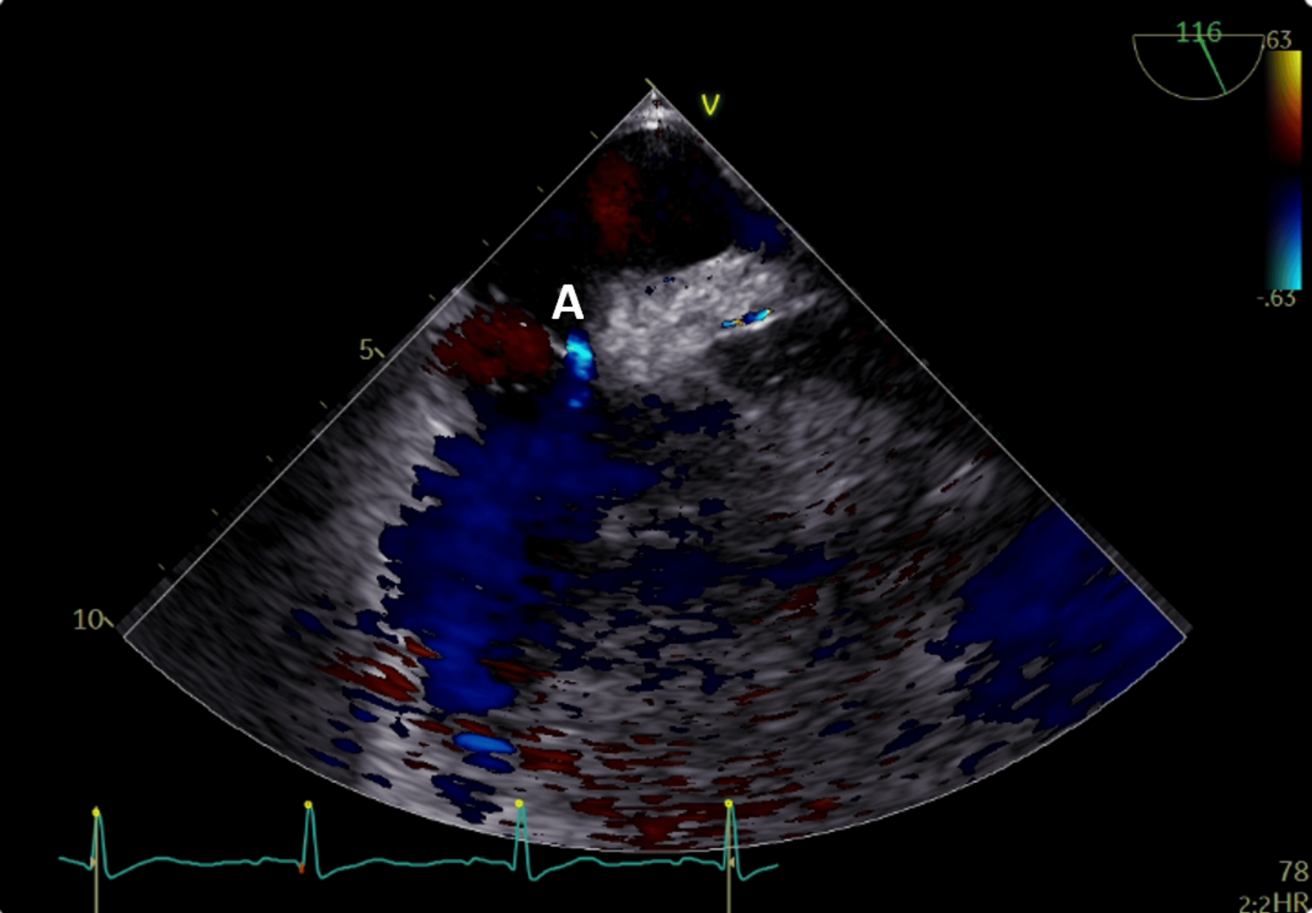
Transesophageal echocardiogram with color Doppler showing the patent foramen ovale (A).
